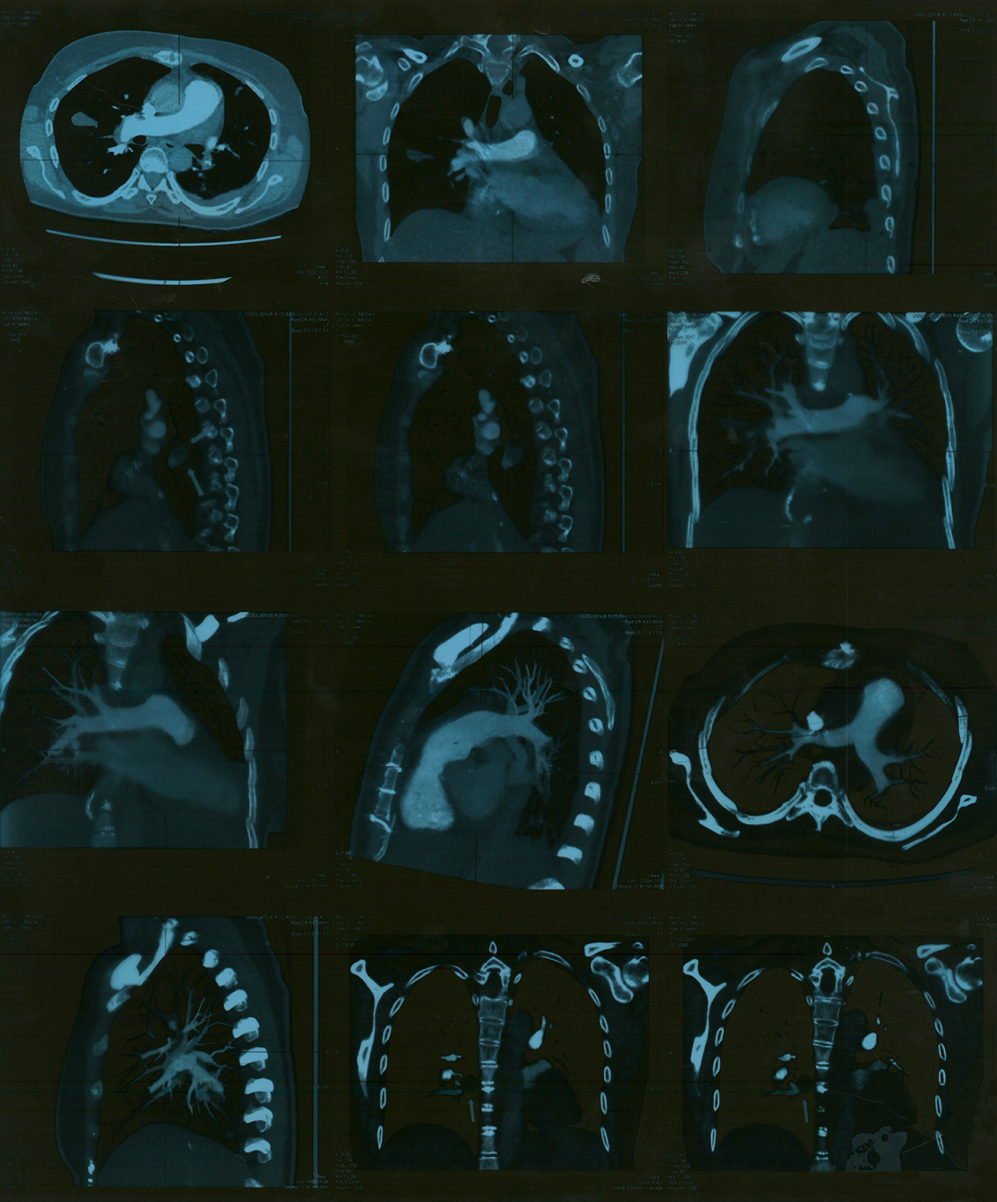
MSCT of coronary blood vessels with massive thromboembolism at the bifurcation of the right pulmonary artery. MSCT, multi-slice CT.
